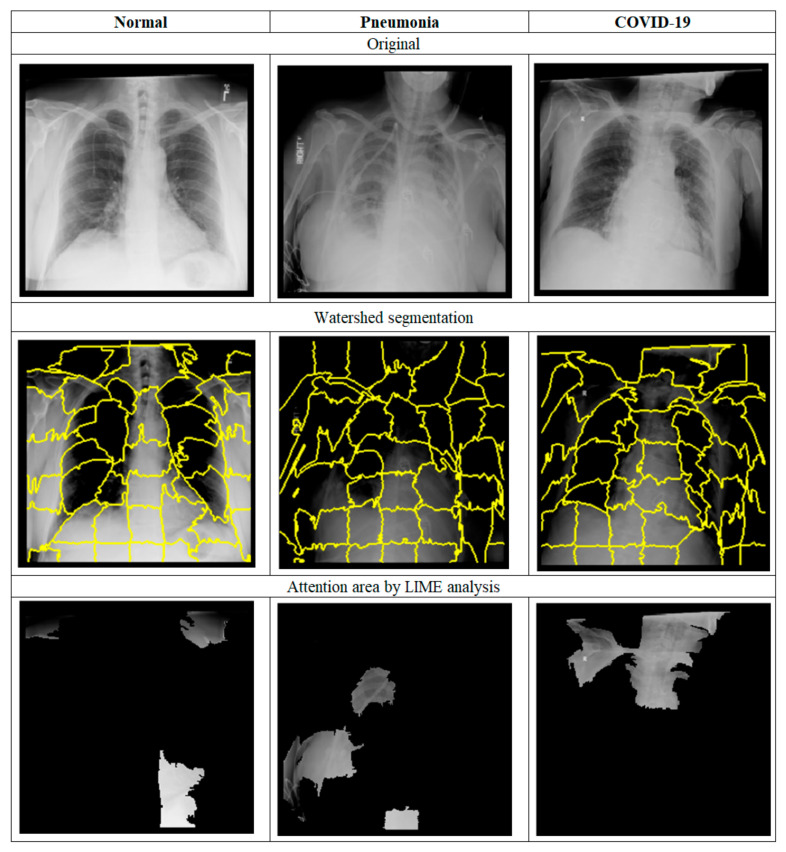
Images for the original Chest X-ray, watershed segmentation, and the results after the LIME analysis.
